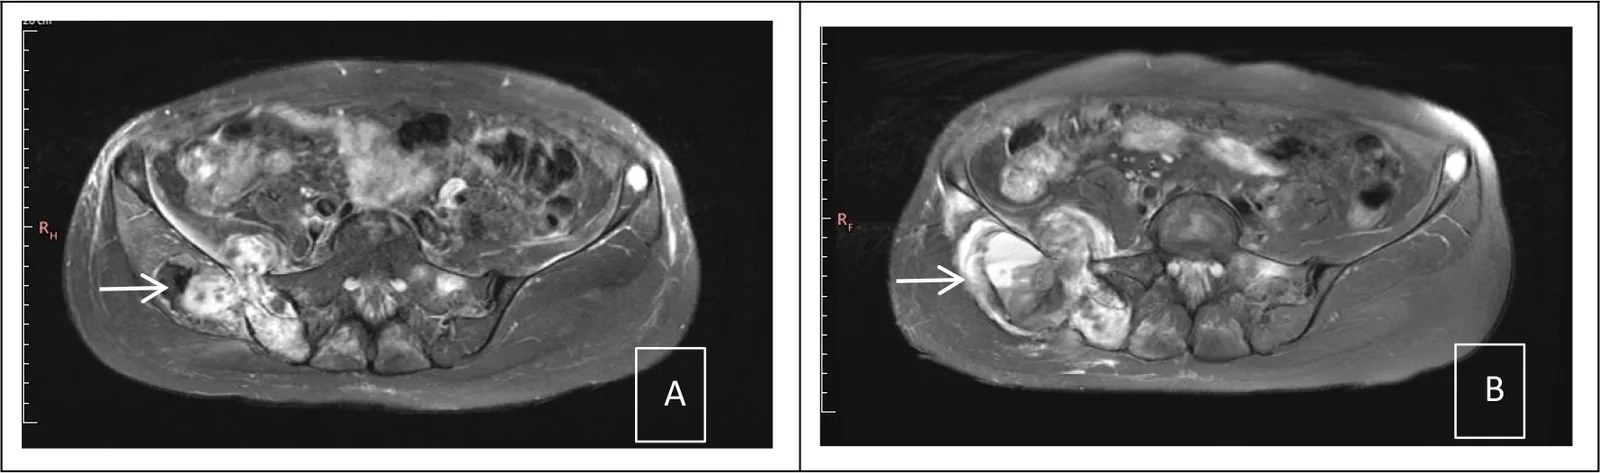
Comparison of initial and follow-up MRI. Axial STIR images of the pelvis. (A) Image at admission shows bone destruction and a surrounding soft tissue mass in the right ilium (arrows). (B) Image from the 21-day follow-up shows interval enlargement of the lesion (arrows).
